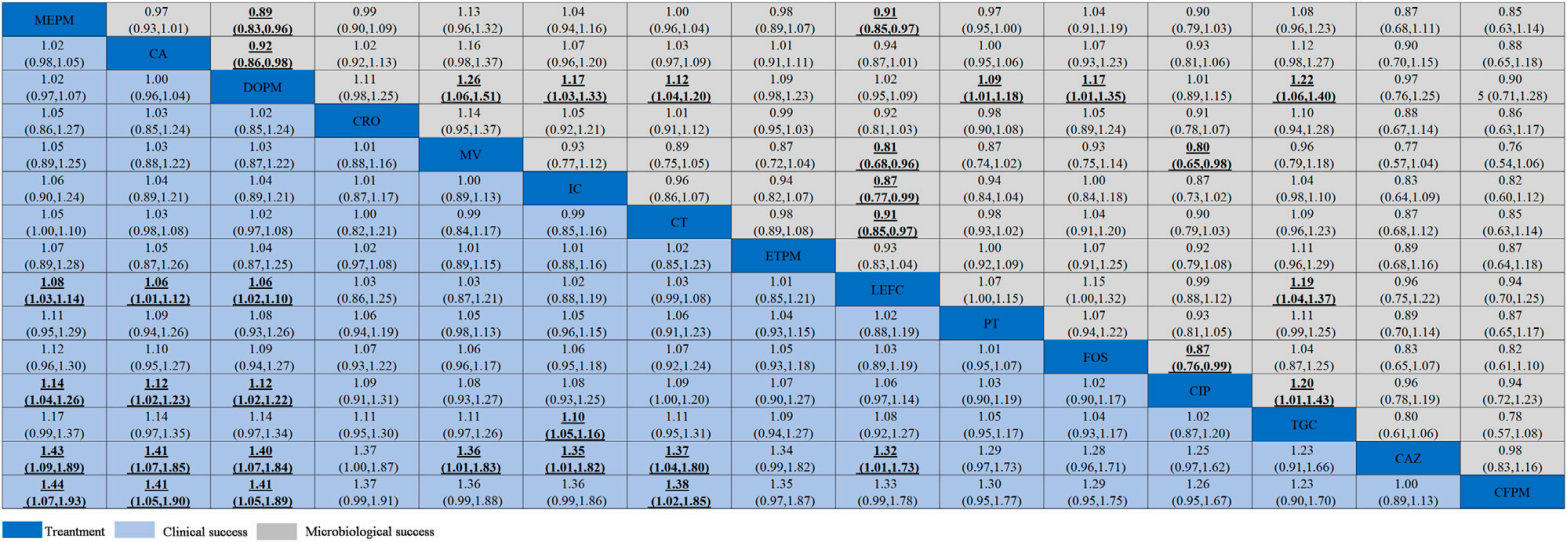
Clinical success and microbiological success of interventions in the treatment of *Enterobacteriaceae* infections. Antibiotics were sorted in the order of decreasing clinical cure rate. The clinical success results were compared from left to right, and the microbiological success results should be read from right to left (the results were expressed by relative risk with 95% confidence interval). Significant results were shown in bold and underlined. CA, ceftazidime/avibactam; MV, meropenem/vaborbactam; MEPM, meropenem; DOPM, doripenem; IC, imipenem/cilastatin; CAZ, ceftazidime; CFPM, cefepime; CIP, ciprofloxacin; PT, piperacillin/tazobactam; ETPM, ertapenem; LEFC, levofloxacin; FOS, fosfomycin; CT, ceftolozane/tazobactam; TGC, tigecycline; CRO, ceftriaxone.
